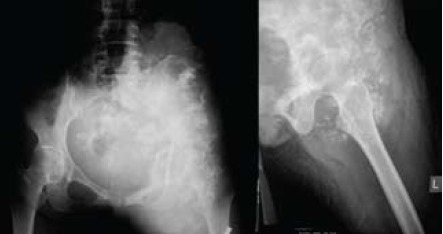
Fig. 3: MRI scan of pelvis showing pelvic mass within pelvic cavity extending to left thigh F3:
